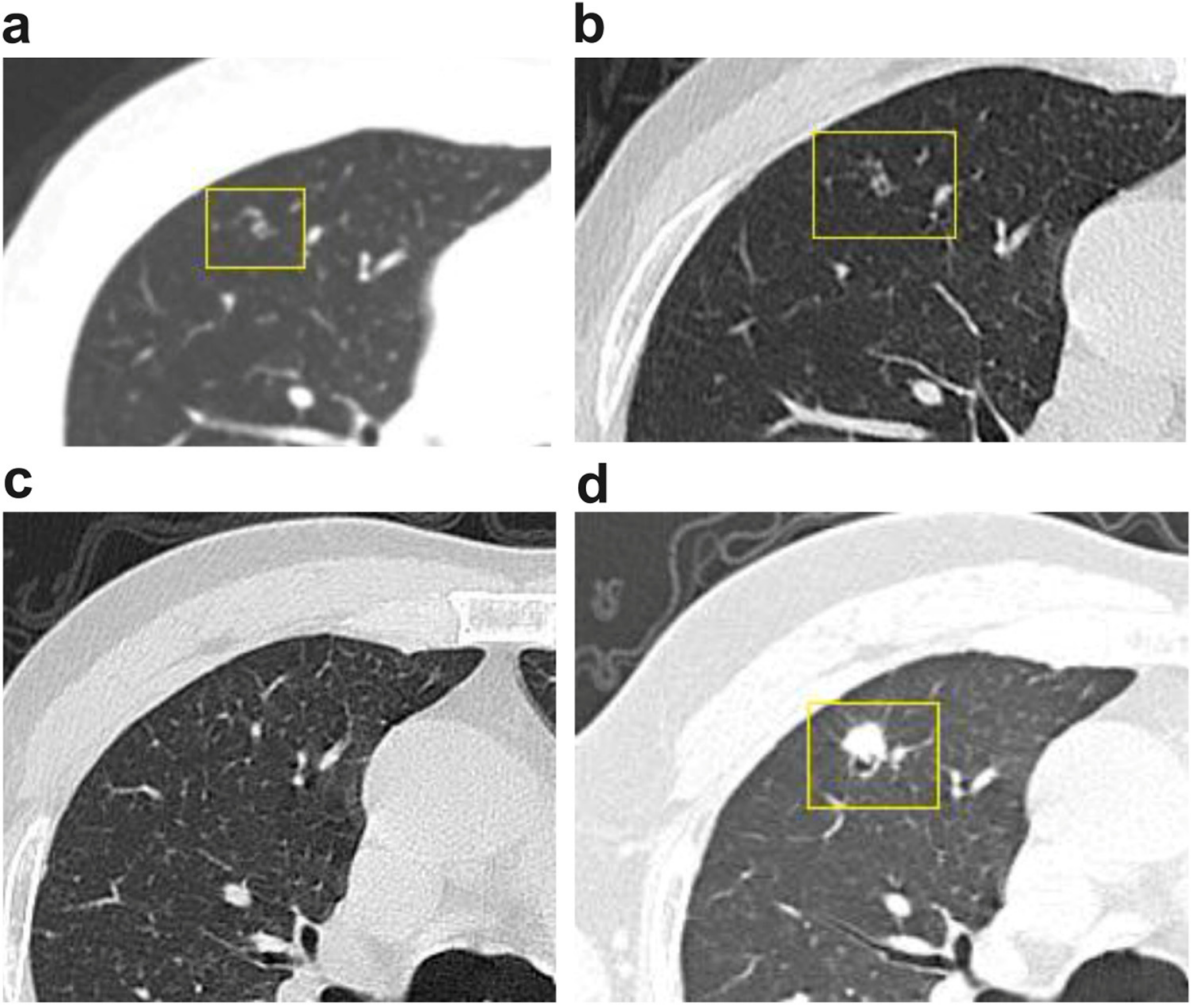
CT scan showing the presence of a cystic airspace in the right upper lobe of the lung on November 18, 2020, (a) and December 17, 2020 (b), the disappearance of the cysts on April 7, 2021 (c), and the presence of a 10-mm-diameter mass with a cystic airspace on April 5, 2024 (d).
